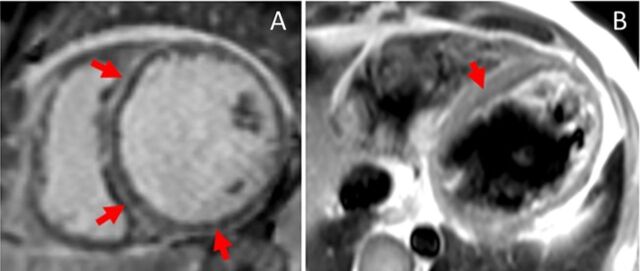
Case 2: Cardiac magnetic resonance imaging shows (A) contiguous mesocardial and epicardial late gadolinium enhancement in a ring-like distribution (arrows), and (B) matched hyperintensity on T2-weighted double inversion recovery, indicating fat infiltration.
